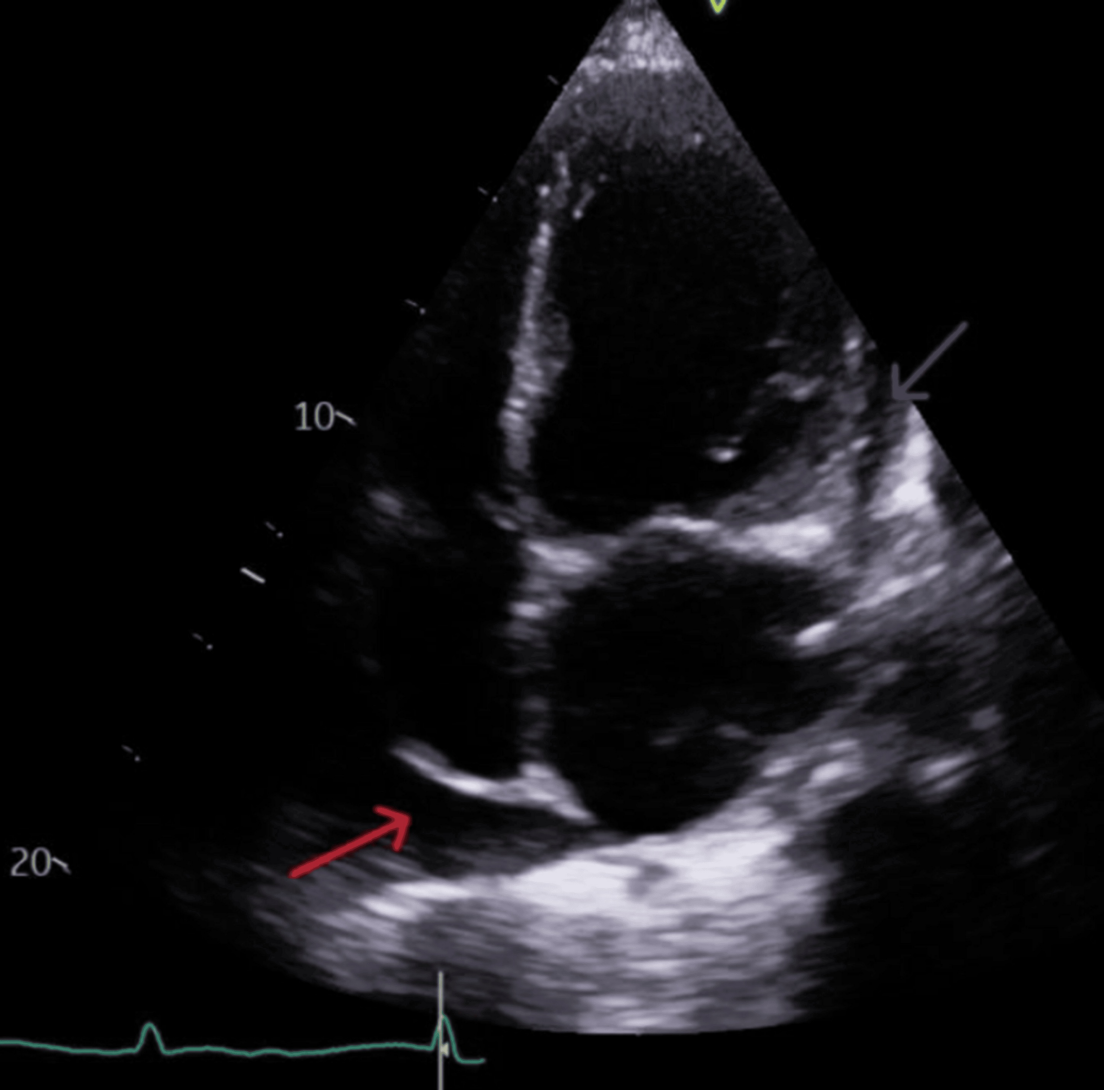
Echocardiogram: Modified apical four-chamber view. The red arrow depicts around 1.7 cm pericardial fluid collection around the right atrium (RA); the purple arrow shows smaller rim of pericardial collection around the left ventricle (LV). This all points towards evidence of polyserositis and reactive pericardial effusion.
